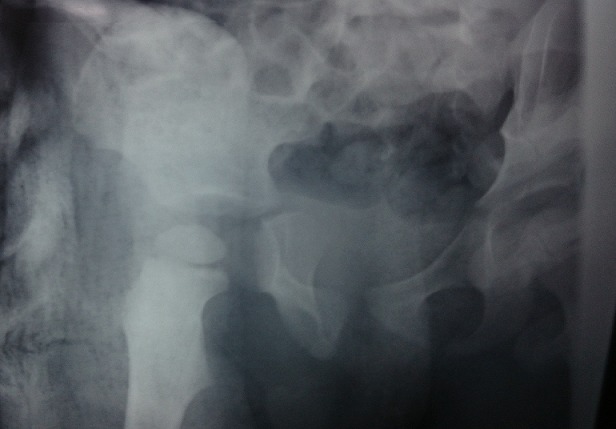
Post-reduction control radiography
